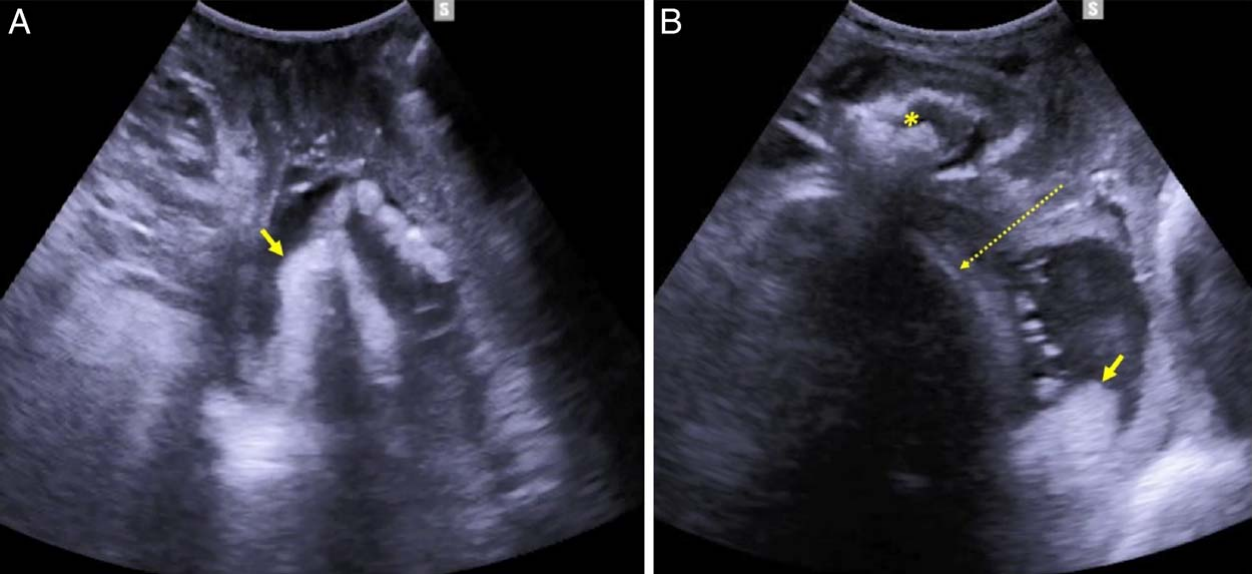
Transperineal ultrasound demonstration of compound hand-cephalic presentation. A, On the axial plane the hand (arrow) is seen preceding the fetal head in the birth canal. B, Midsagittal view demonstrating the relation between the hand (arrow), the pubic symphysis (*), and the fetal head (dotted arrow).
